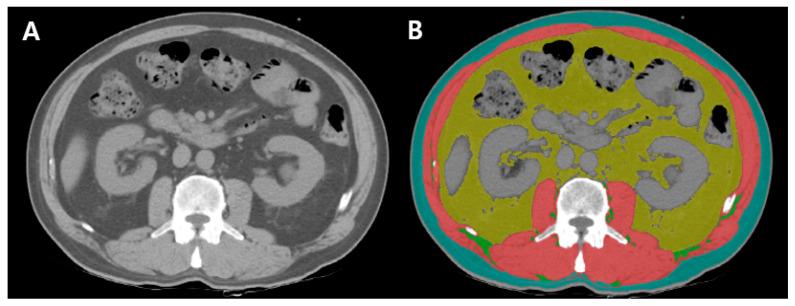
Cross-sectional CT images at the level of L3. (A) Unenhanced image using Picture Archiving and Communications System (B) body composition parameters classified by color-coded distribution areas using AutoMATiCA (Red, skeletal muscle area; yellow, VAT area; blue, SAT area; green, IMAT area [20].
